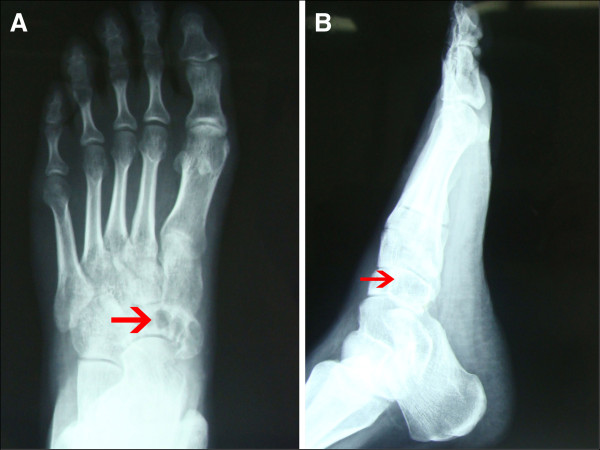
Radiographs of the patient’s left foot. (A) Anteroposterior view shows an expansile lesion of the navicular bone and (B) Lateral view shows thinning of the navicular cortex.
